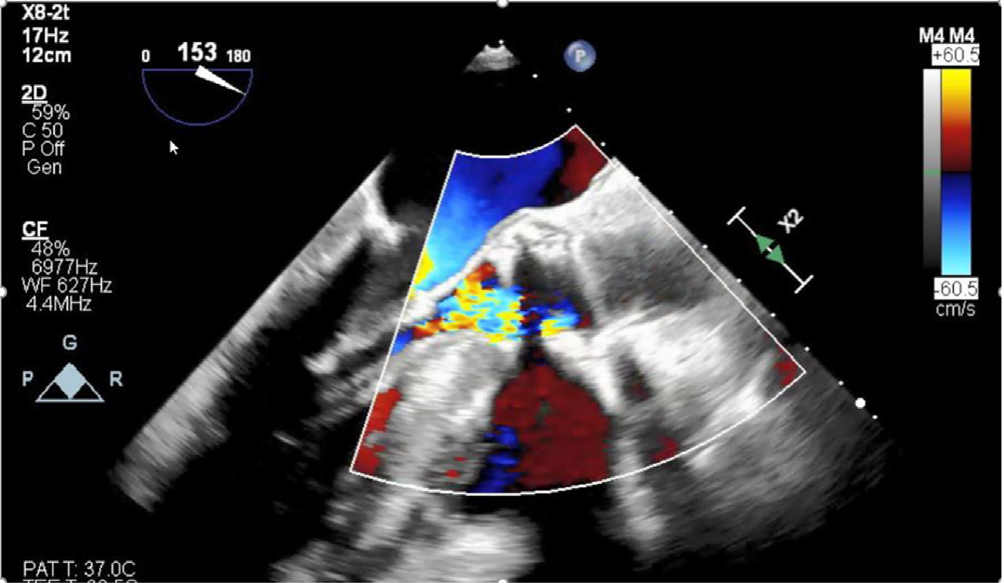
Transesophageal echocardiogram long axis view showing frozen leaflet causing severe AR. Abbreviation: AR, aortic regurgitation.
